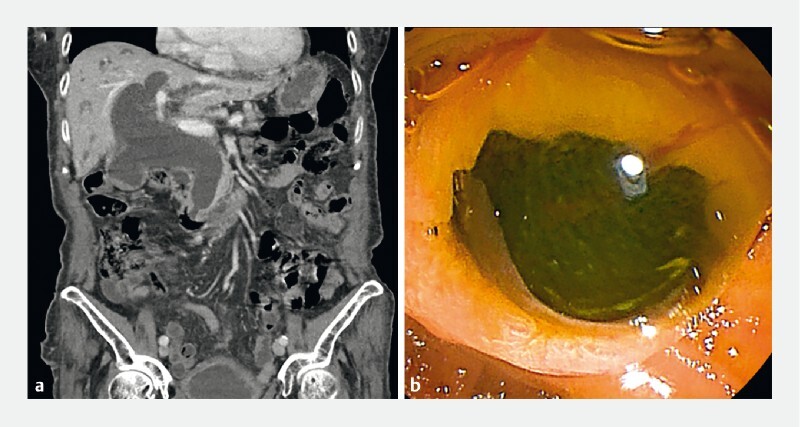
Initial abdominal computed tomography (CT) and endoscopic findings.
